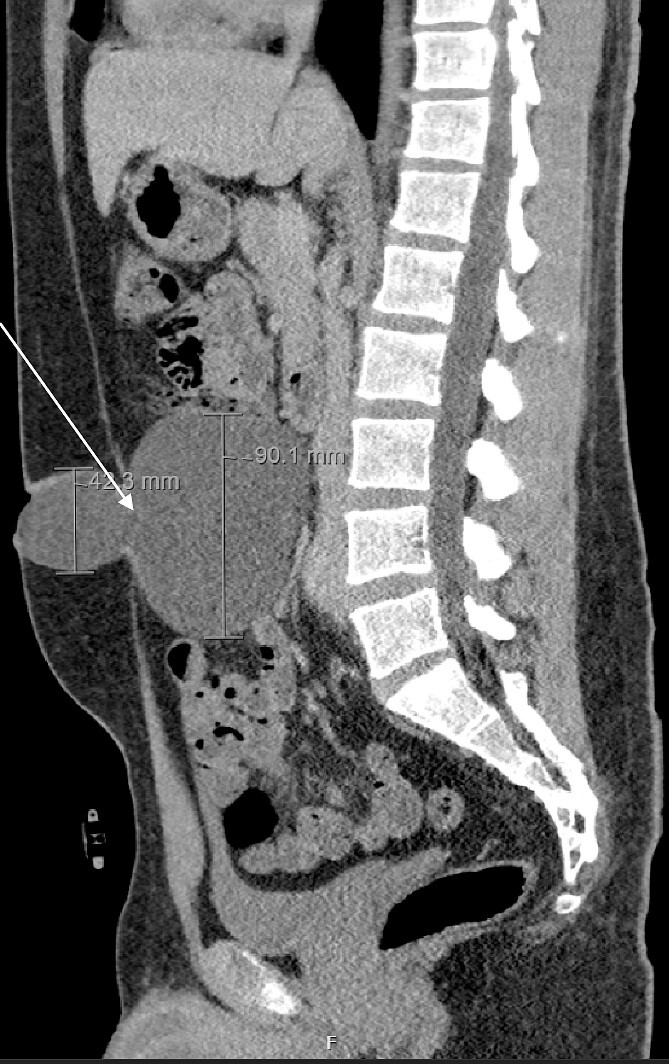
CT scan in sagittal cross-section demonstrating PIC.
